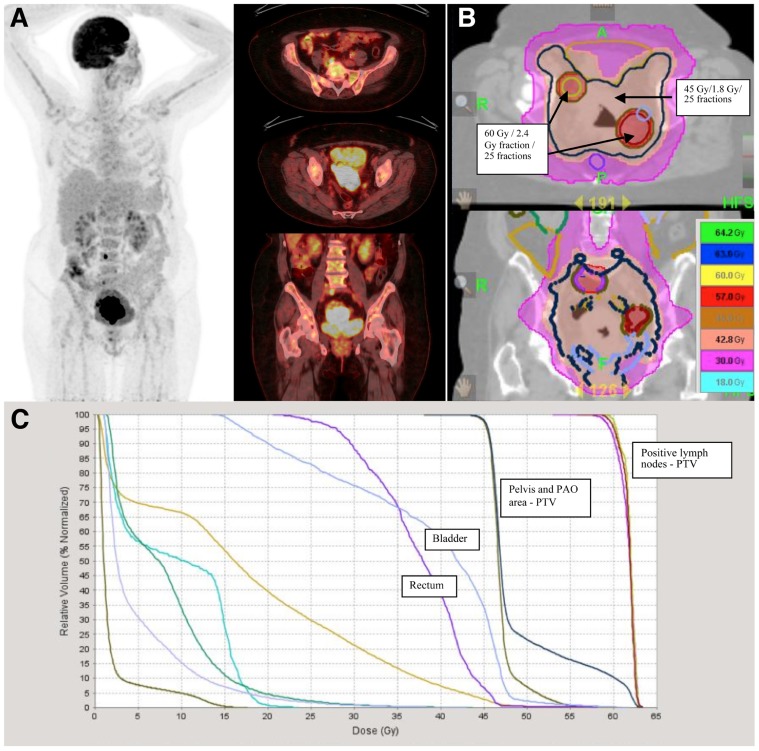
A 62-year-old lady with FIGO stage IIB cervix cancer and positive pelvic lymph nodes was treated with cisplatin based chemotherapy and radiotherapy. (A) Positive lymph nodes are delineated based on FDG-PET/CT uptake and treated with 60 Gy in 2.4 Gy per fraction in 25 fractions. (B) Radiotherapy was delivered with helical tomotherapy. Pelvis and para-aortic areas were treated with 45 Gy in 25 fractions of 1.8 Gy. (C) Scale dose banding shows the 95% of the dose. The tumor boost was delivered with MRI-guided brachytherapy in four fractions of 7 Gy.
